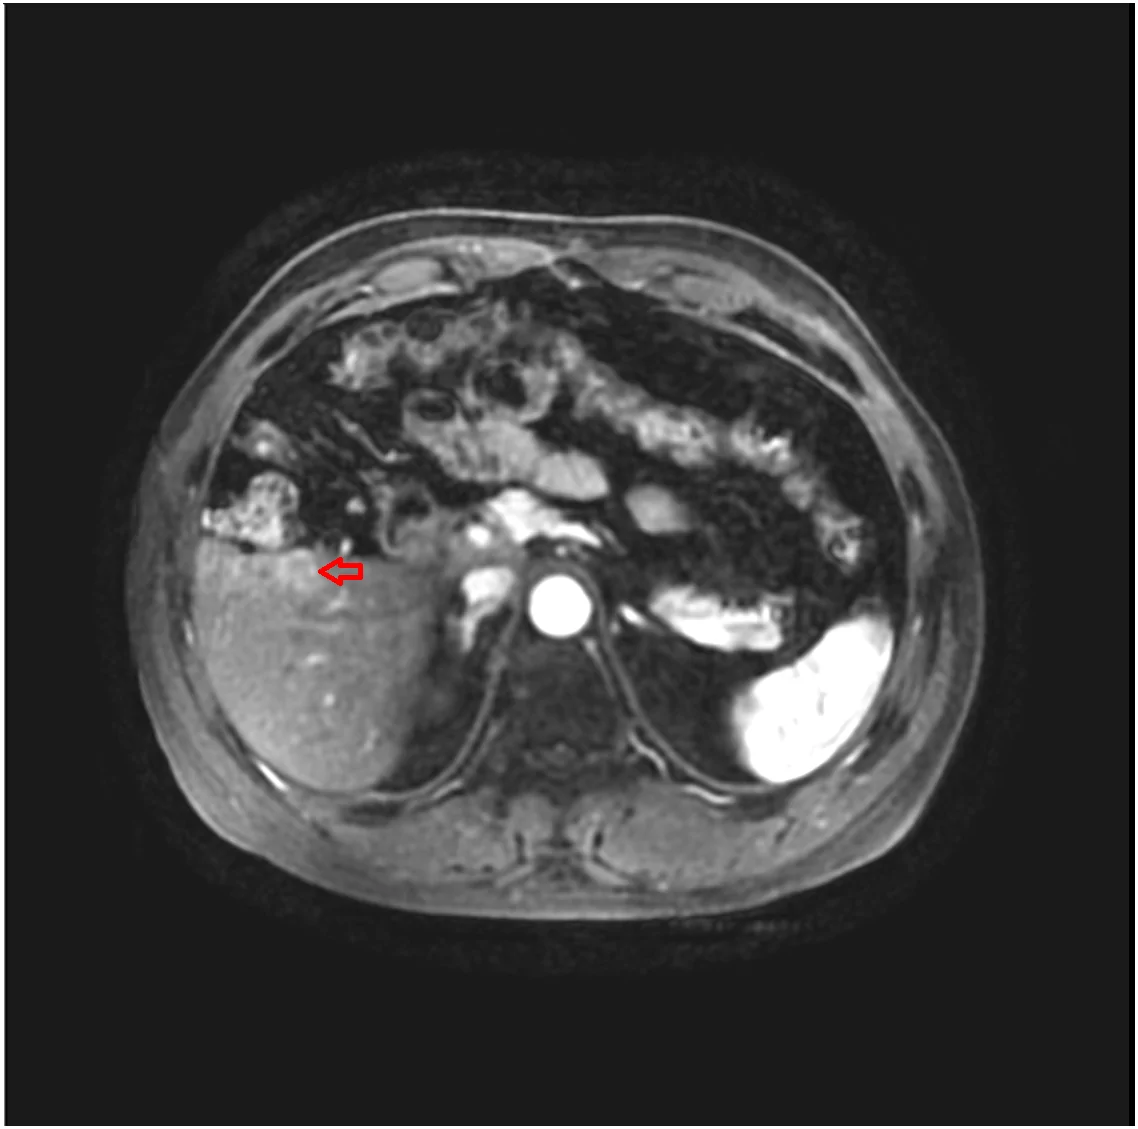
Postoperative MRI images of the patient, showing a new circularly enhanced lesion at the margin of the surgical area in the right lobe of the liver, suggestive of metastasis.
